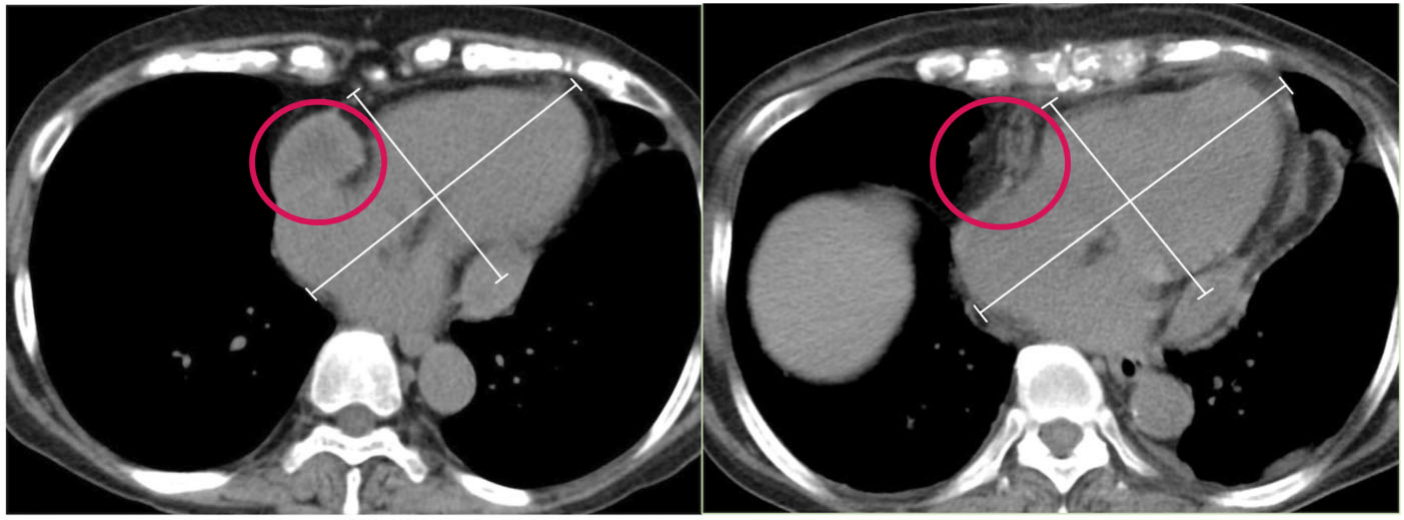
(Left) Computed tomography shows circumferential thickening of the pericardium. The heart measured 131 mm × 93 mm. (Right) The thickened pericardium was removed, resulting in the enlargement of the heart (circle). The heart dilated to 143 mm × 102 mm.
